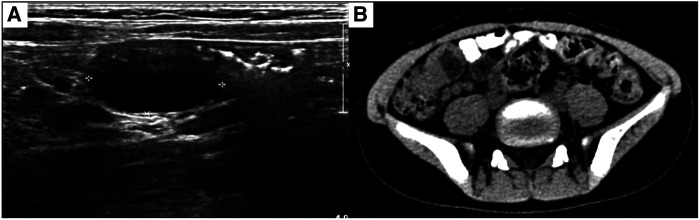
(A) Ultrasound revealing a cystic mass measuring 20 × 17 × 12 mm at the end of the ileum wall. (B) Abdominal CT showing two round cystic low-density shadows in the right lower abdomen, with sizes of 25.9 × 22.5 mm and 19.1 × 14.1 mm, respectively. The lesions are close to the bowel and show compression. ▴: Lesion in ileocecal junction; ▾: Lesion 5 cm from ileocecal junction.
